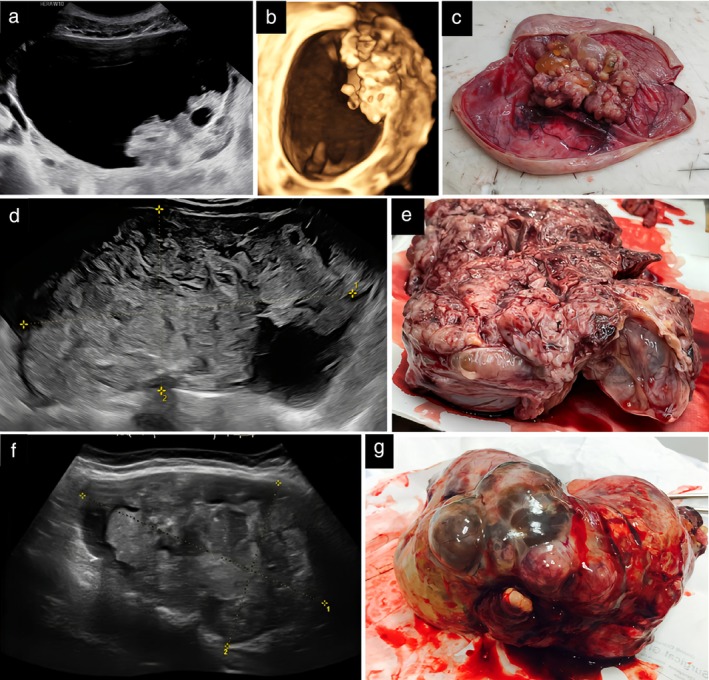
Grayscale ultrasound images (a,d,f), three‐dimensional rendering (b) and corresponding macroscopic appearance (c,e,g) of three ovarian immature teratomas: (a–c) tumor 1, (d,e) tumor 2 and (f,g) tumor 3.
